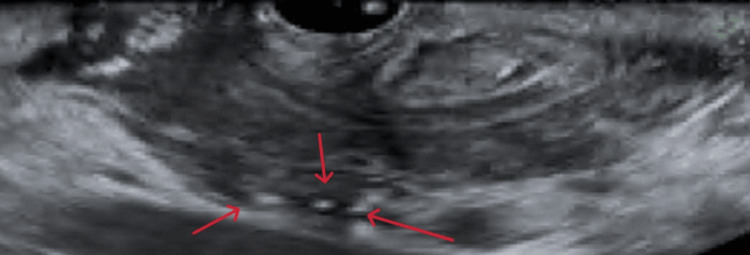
EUS showing multiple calcifications in the pancreas (red arrow) EUS: Endoscopic ultrasound
